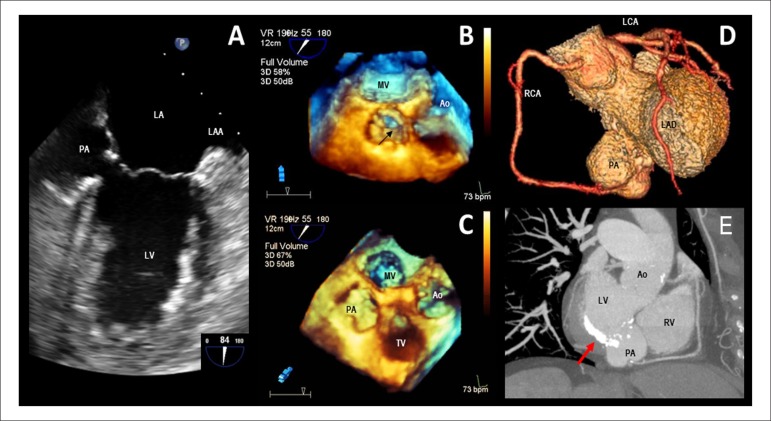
A. Transesophageal echocardiography (TEE) showing a pulsatile perivalvular echo-free
